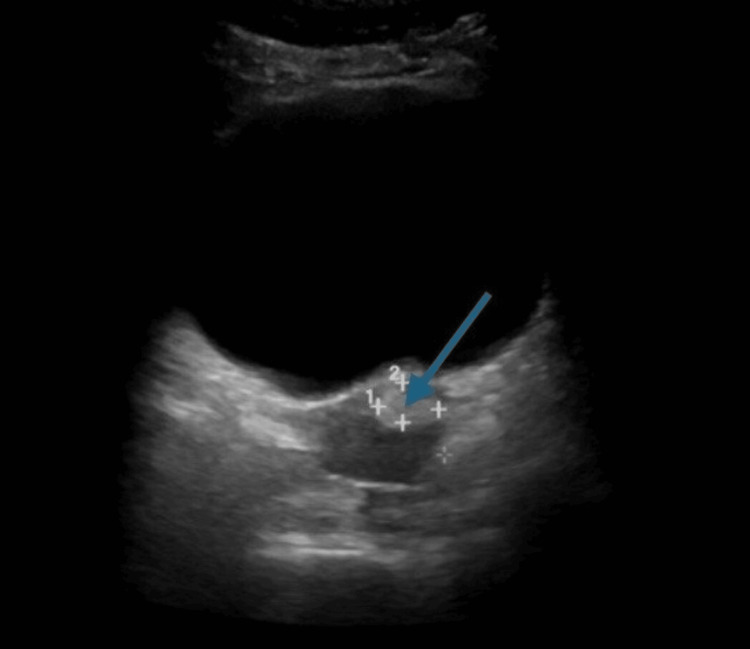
Ultrasound showing a hyperechoic lesion in the anterior myometrial wall (see arrowhead).
